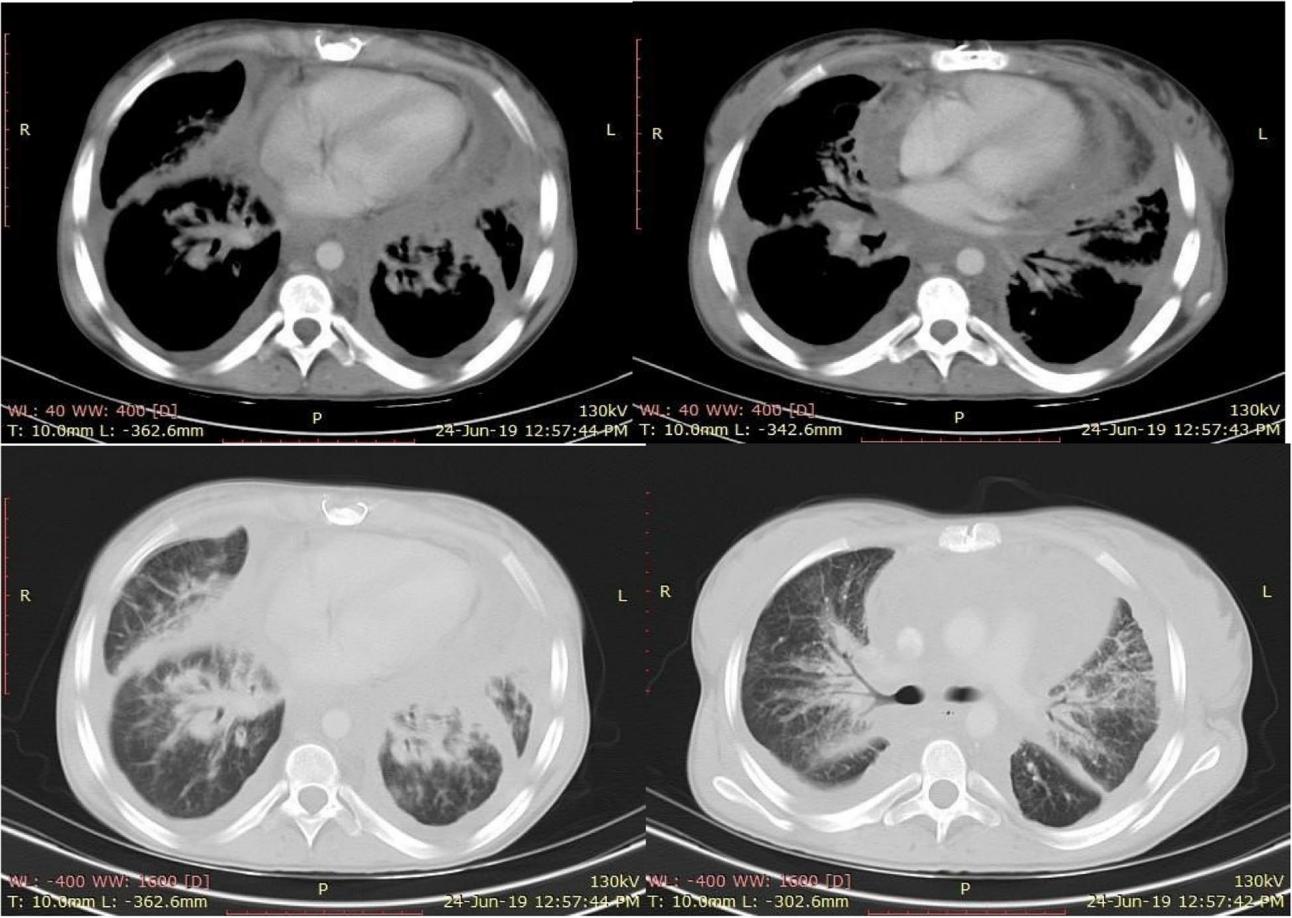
CT chest showing diffuse mediastinal infiltrative soft tissue lesion, pericardial thickening with effusion, bilateral diffuse thickening of central and peripheral pulmonary interstitium
